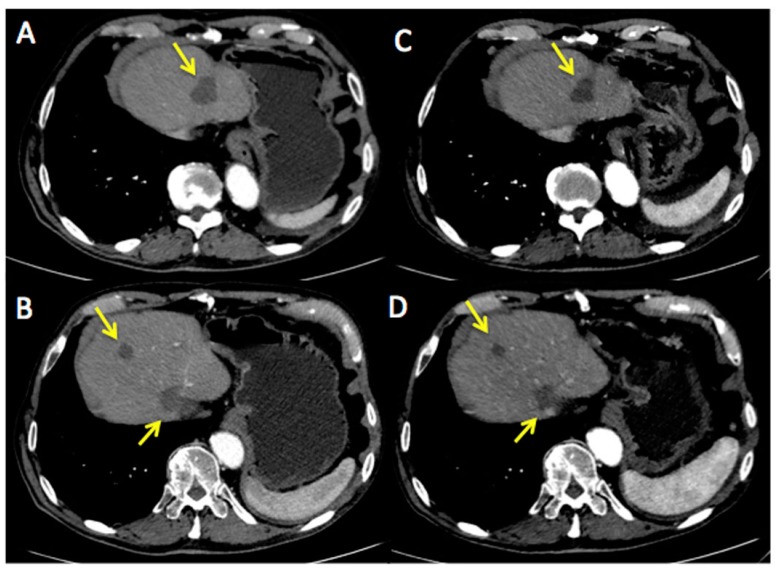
A 71-year-old male affected by colorectal cancer with liver metastasis, subjected to 6 cycles of Bevacizumab-based chemotherapy and 10 hyperthermia sessions on the abdomen as second-line. Baseline Multidetector Contrast Enhancement Computed Tomography (MDCT) (A,B) showed metastasis in the II and VIII segment of the liver and caudate lobe (maximum diameter: 47 mm, yellow arrows). Timepoint-1 MDCT (C,D) evaluation demonstrated stable size of liver metastasis (maximum diameter: 44 mm, yellow arrows). According to mRECIST, patient was classified as SD. Ca19.9 and Carcino-Embryonic Antigen (CEA) values evaluated at baseline were 99 UI/mL and 65 ng/mL, respectively; while Ca19.9 and CEA values evaluated at timepoint-1 were 81 UI/mL and 60 ng/mL, respectively. Side effects reported were limited to nausea and mild position-related pain during HT sessions.
